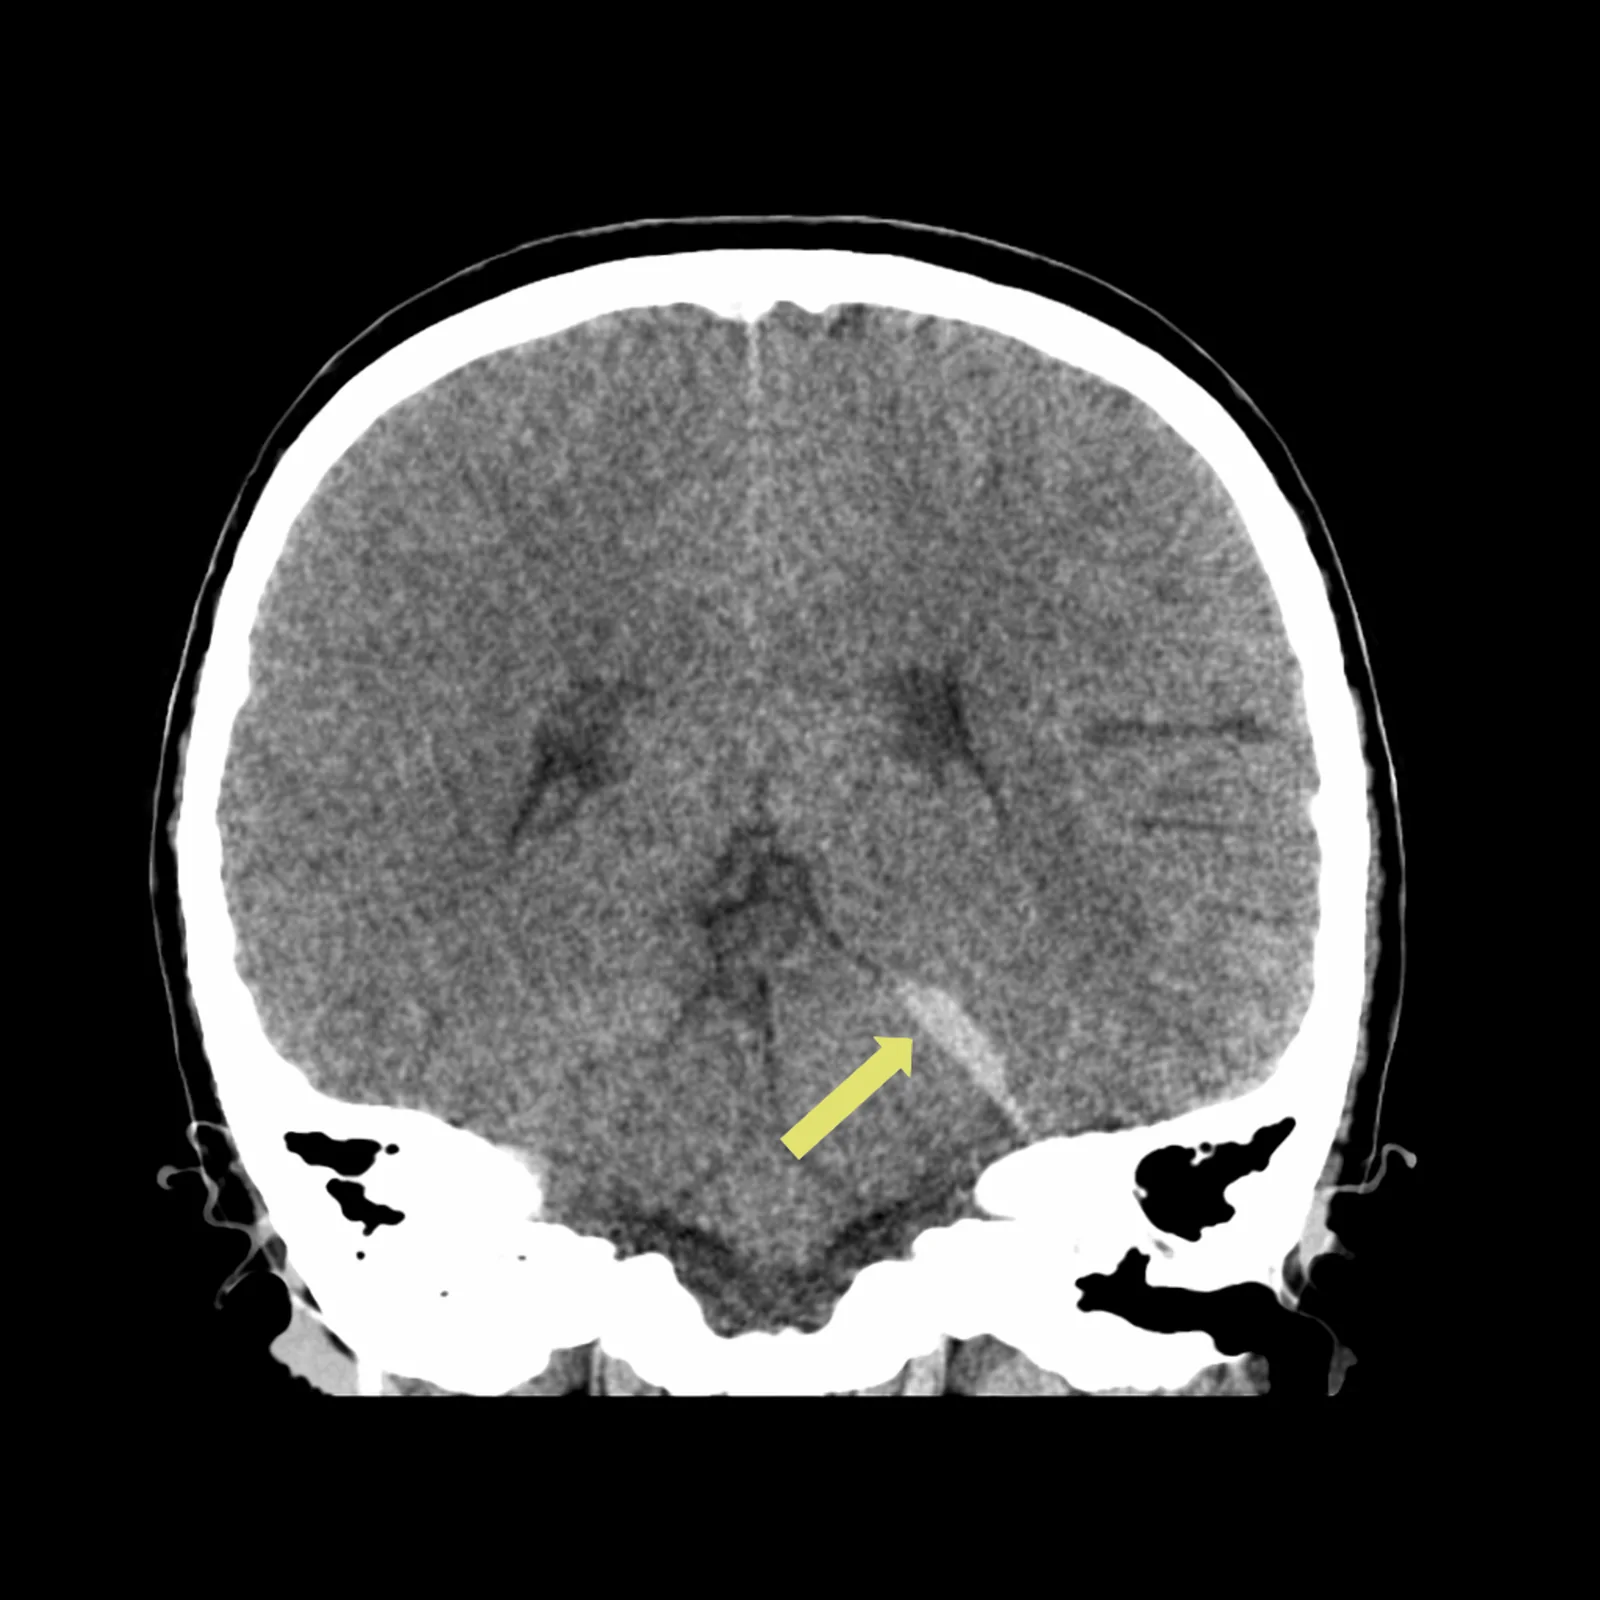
Coronal noncontrast CT image showing the same left high parietal cortical-subcortical wedge-shaped abnormality. Noncontrast CT of the brain demonstrates a left high parietal cortical-subcortical wedge-shaped area containing subtle linear hyperdensities (arrow). No other areas of acute hemorrhagic blood products are identified. There are no additional abnormal areas of increased or decreased attenuation. The ventricular system is normal in size and configuration. No intra-axial or extra-axial collections or space-occupying lesions are seen. There is no evidence of mass effect or midline shift. The midbrain and posterior fossa structures appear unremarkable.
